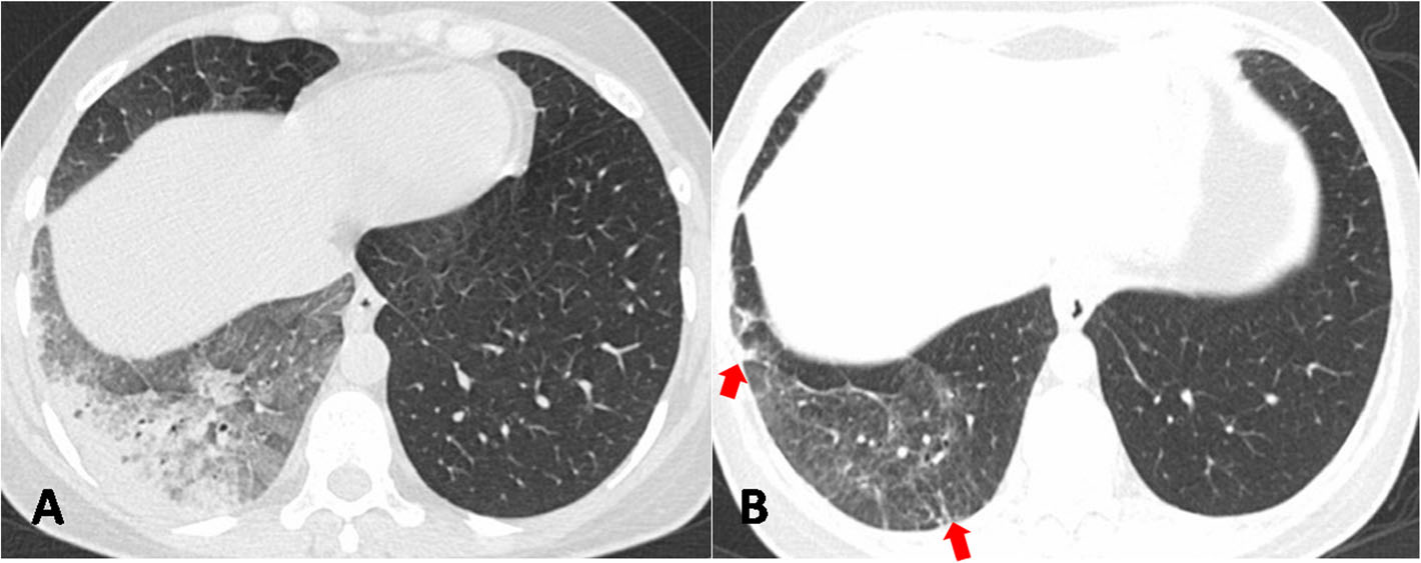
Axial chest CT image in lung window settings of a 36-year-old COVID-19 patient showing a mixed GGO and consolidation in right lower lobe at admission (a). Follow-up CT performed 92 days later reveals residual GGO with subpleural parenchymal bands (red arrows) (b)
